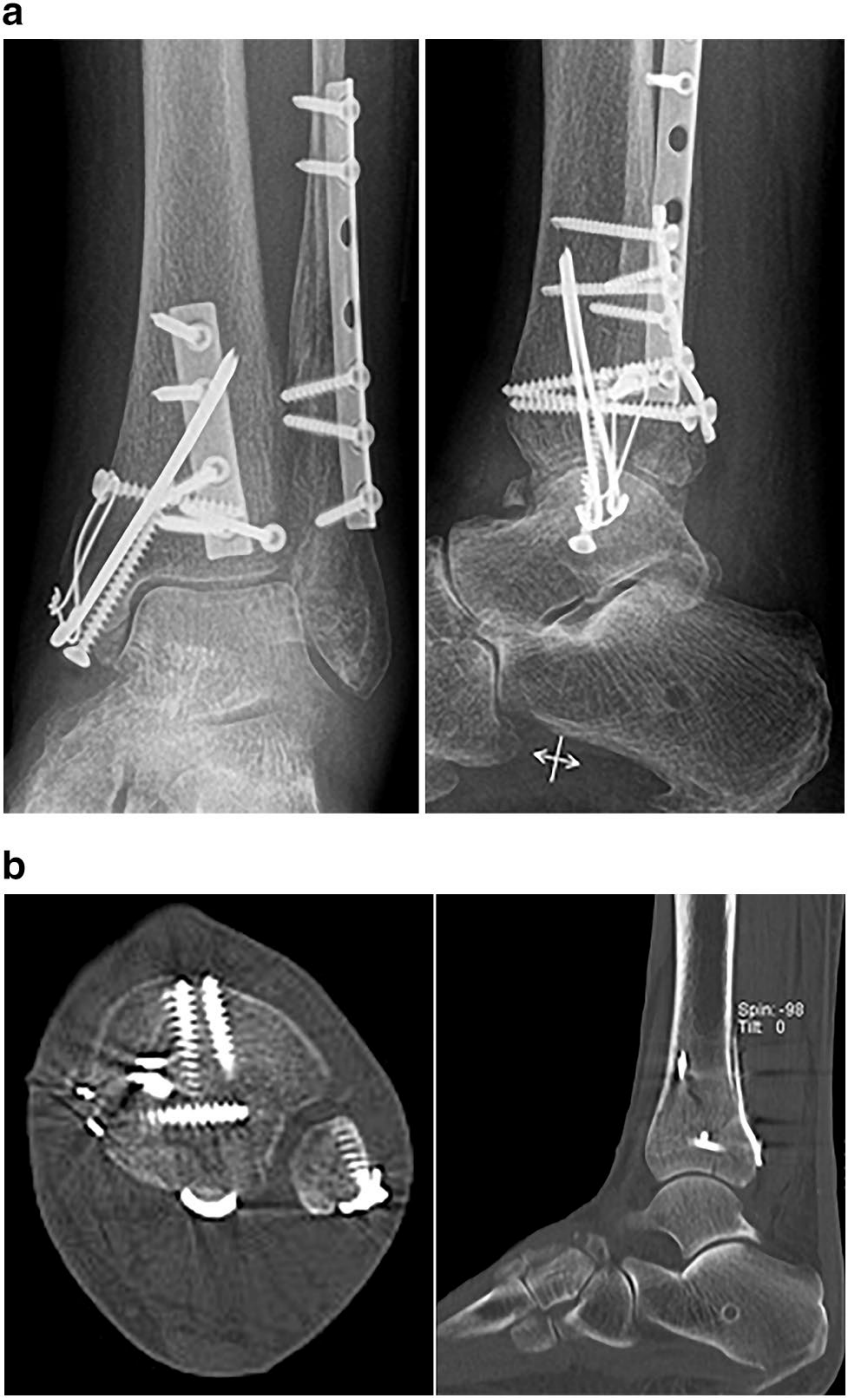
a Postoperative anteroposterior and lateral radiographs and b axial and sagittal CT scans after open reduction and posterior antiglide plate fixation of the distal tibia and fibula via a posterolateral approach and medial malleolar fixation via a medial approach all with the patient in prone position (same patient as in Fig. 1)
